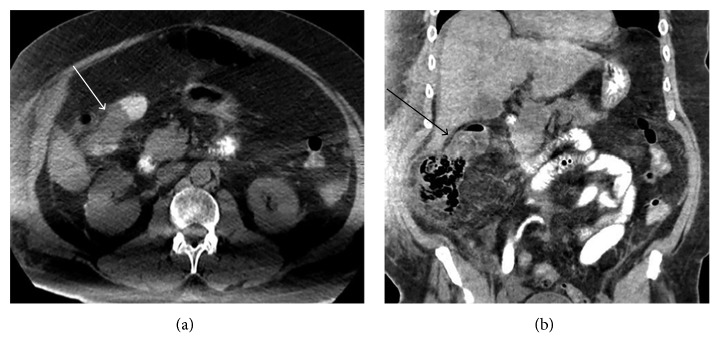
Abdominal computed tomography (CT) demonstrating apple-core mass of the ascending colon.
